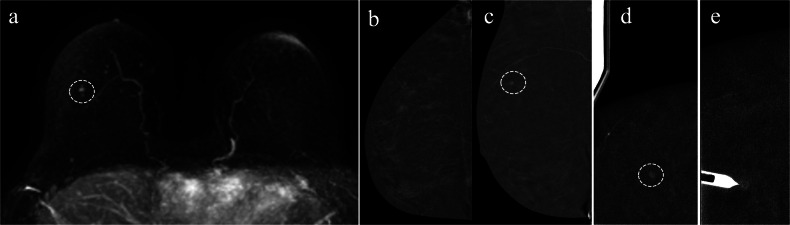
CEM in a 50-year-old woman with a history of left breast cancer and BRCA2 mutation. During follow-up, MRI identified a new 4 mm enhancing focus in the upper outer quadrant (UOQ) of the right breast (dashed circle in (a)), with no correlate on ultrasound. To confirm lesion visibility, a unilateral CEM was performed. Panels (b) and (c) show craniocaudal (CC) and mediolateral (ML) RC images, respectively. The lesion is more conspicuous in the upper quadrants of the ML view (dashed circle in (c)), which was chosen for targeting during a CEM-guided biopsy on a different day (RC scout (d) and pre-fire view (e)). HP reported high-grade triple-negative IDC
